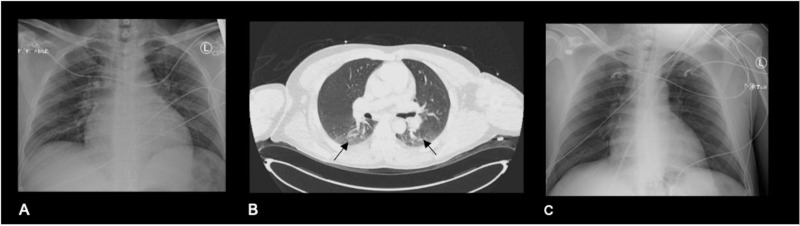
Chest imaging (A) Posteroanterior (PA) chest X-ray on admission demonstrates clear lung. (B) Axial CT chest demonstrates clear lungs with slight atelectasis in the dependent portion of the lungs (black arrows). (C) Chest X-ray on readmission was still clear.
